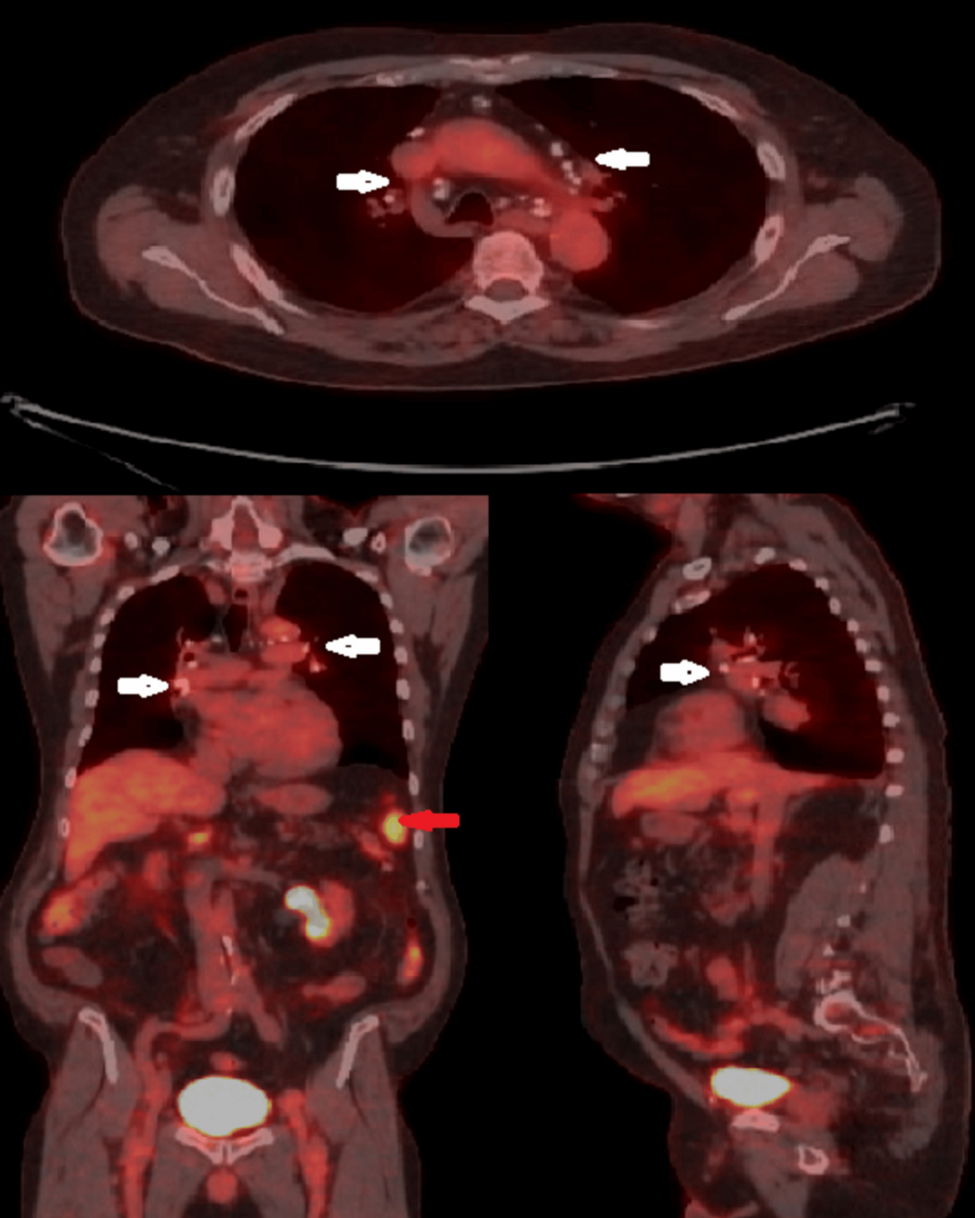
Positron emission tomography-computed tomography demonstrating symmetric calcified mediastinal and bilateral hilar lymph nodes, marked by white arrows, and splenic involvement with diffuse increased fluorodeoxyglucose uptake, marked by red arrow.
